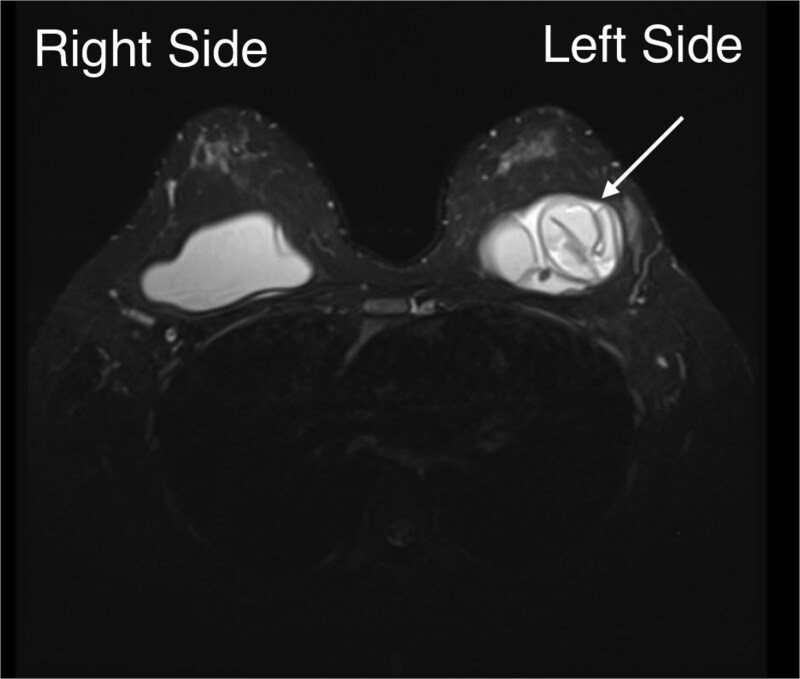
Postpartum breast MRI: The left implant shows the linguine sign represents intracapsular rupture and focal shell discontinuity in the upper half of the implant, with an associated subcapsular/peri-implant fluid collection measuring up to 24 mm. The right breast one shows an intact implant without shell discontinuity or peri-implant fluid collection.
